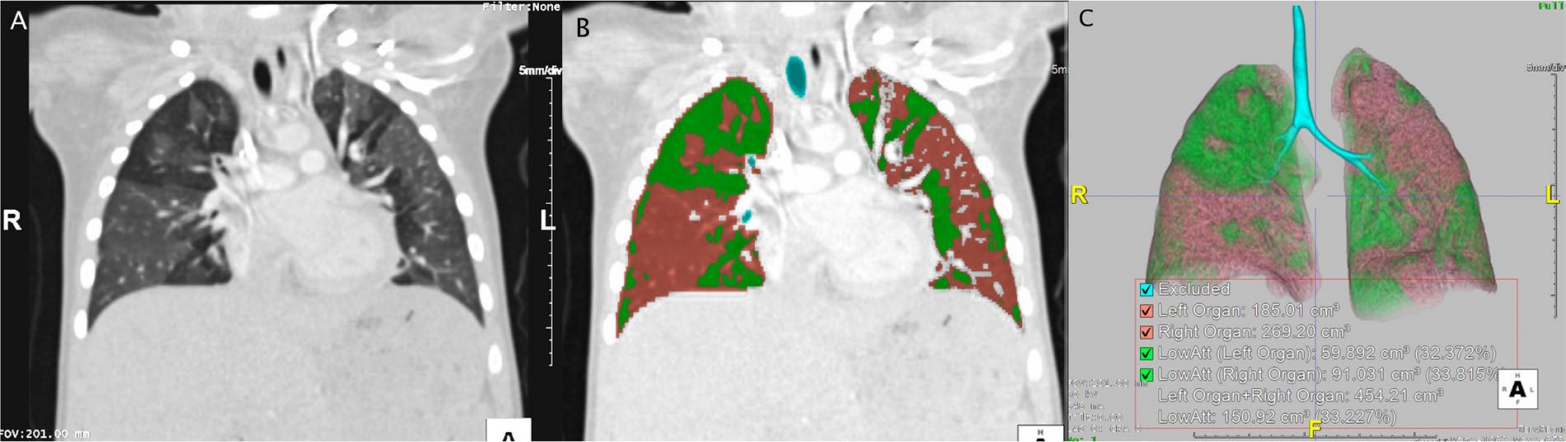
Quantitative chest CT assessment in a 21-month-old boy with post-infectious bronchiolitis obliterans. (A) Coronal image shows differing multifocal attenuation areas in both lungs from air-trapping and normal aeration. Normal lung attenuation value (HUn) was -568.4 HU and air-trapping lung attenuation (HUa) was -836.8 HU in this patient. (B) Both lungs were segmented computationally by applying the cutoff attenuation value (HUc, -702.6 HU for this patient). The same coronal image in part (A) shows the air-trapping areas in green, the normally aerated lung in orange, and the excluded airway in blue. (C) The 3D color image shows the calculated total lung volume (454 ml), air-trapping lung volume (151 ml), and air-trapping lung volume percentage (33.2%) in this patient.
